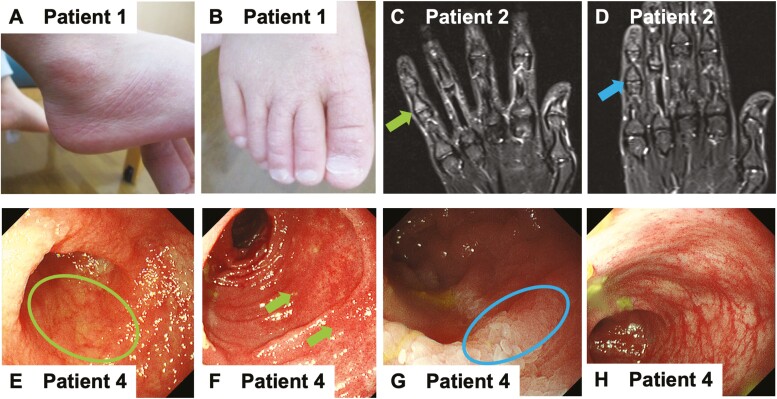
Clinical images of the patients. (A and B) Images of the right ankle and toes of patient 1 before the administration of tofacitinib. The patient had an atopic dermatitis-like skin rash. (C and D) Magnetic resonance imaging findings of the right hand of patient 2 before and after the initiation of tofacitinib treatment. (C) Findings before starting tofacitinib treatment with an enhanced T2-weighted short tau inversion recovery image around the proximal interpharangeal joint of the little finger which is suggestive of arthritis, including bone marrow edema and contrast effects of the tendon (green arrow). (D) Findings 6 months after initiation of tofacitinib treatment. Abnormal lesions showed improvement (blue arrow). (E–H) Endoscopic images of patient 4 before and after the administration of ruxolitinib. (E and G) Terminal ileum; (F and H) Descending colon; (E and F) Before administration of ruxolitinib treatment; (G and H) After 4 months of ruxolitinib treatment. Initially, the ileum showed total villous atrophy (green circle) and the colon showed a decreased vascular pattern with edematous mucosa (green allows); however, after the administration of ruxolitinib these abnormal findings showed improvement (blue circle).
